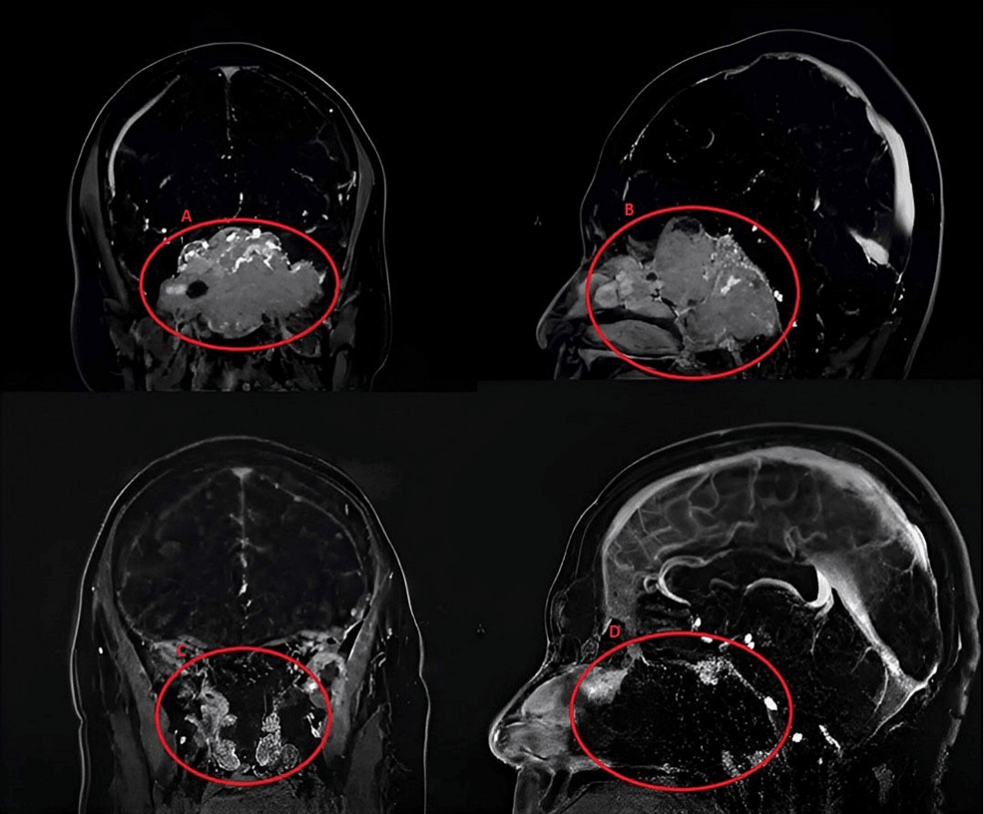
Coronal and sagittal brain MRI axial T1-weight contrast-enhanced showing a large clival tumor (A and B) that was resected in the middle line (C and D).
